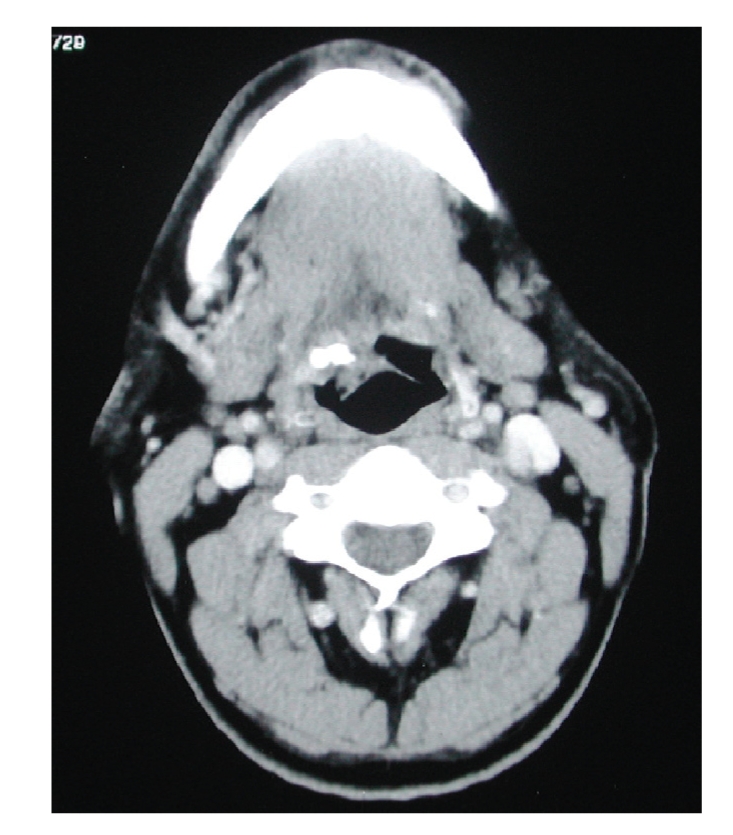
CT scan of the lesion. The lesion can be seen on the right
